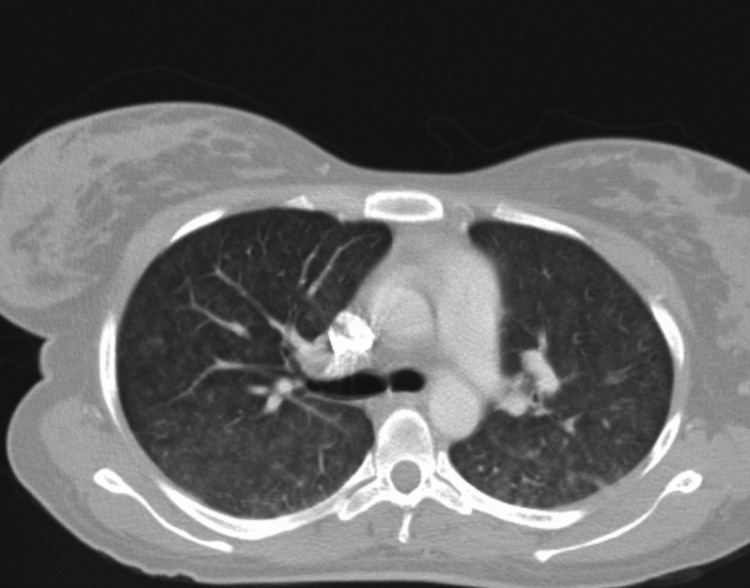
CT Pulmonary Angiography Computed tomography (CT) pulmonary angiography showing ground glass opacities consistent with hypersensitivity pneumonitis.
